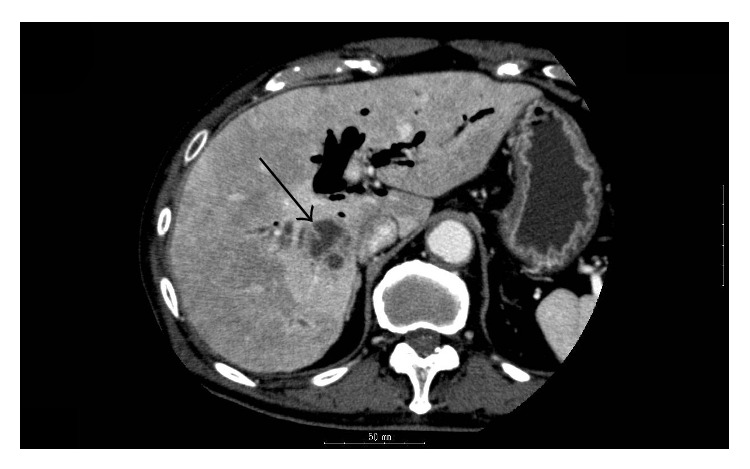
CT scan showing contrast-enhanced lesion in liver (arrow).
